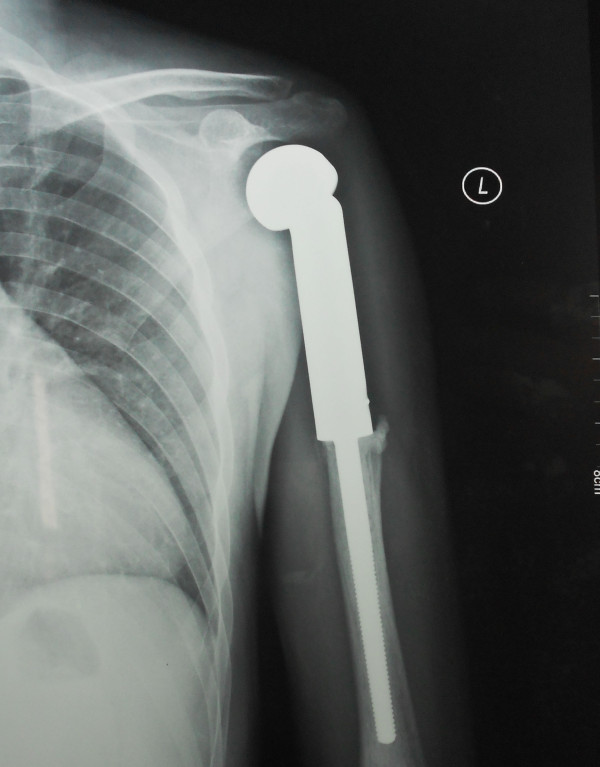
Post operative X-ray (AP view) showing 39 months follow-up of the same patient.
